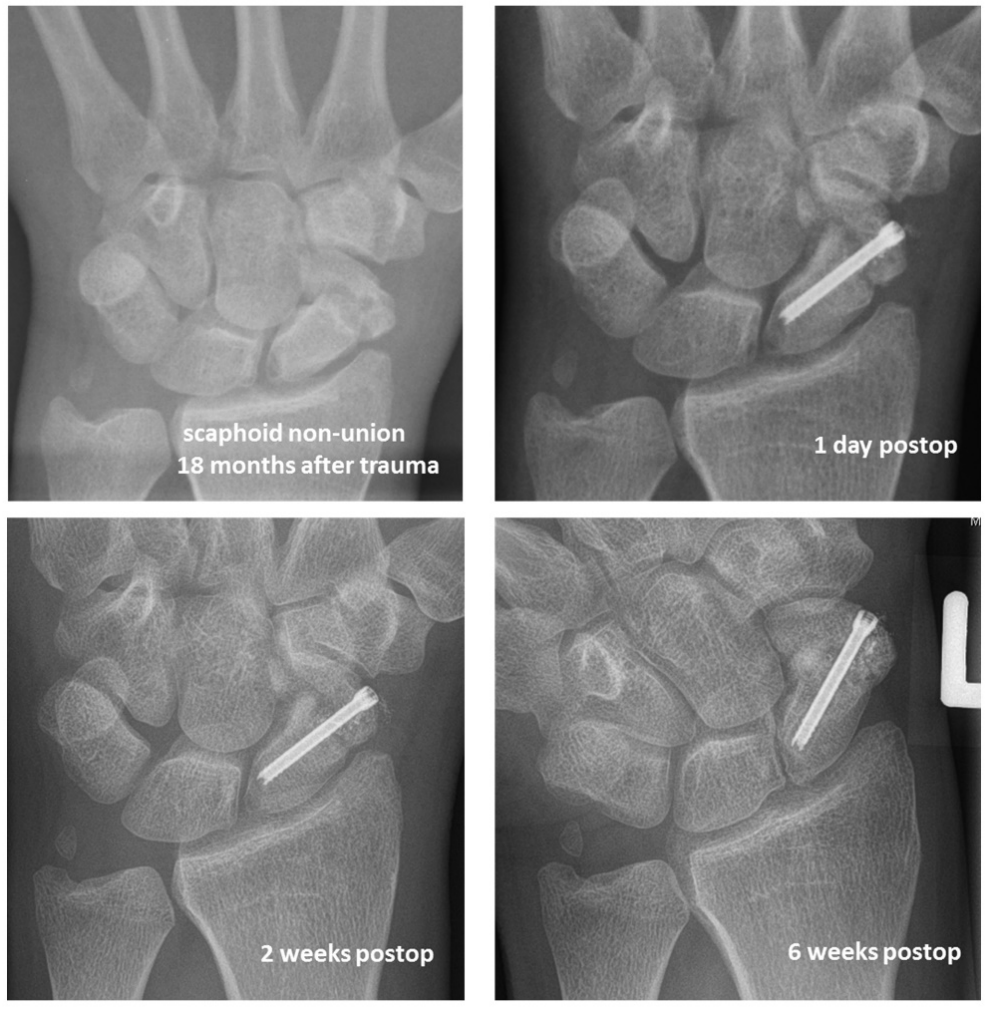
X-ray of an 18 months old scaphoid non-union of a 32 y.o. male treated with a bone graft from the iliac crest and an HCS (Synthes^®^), 6 week post-operative follow-up
